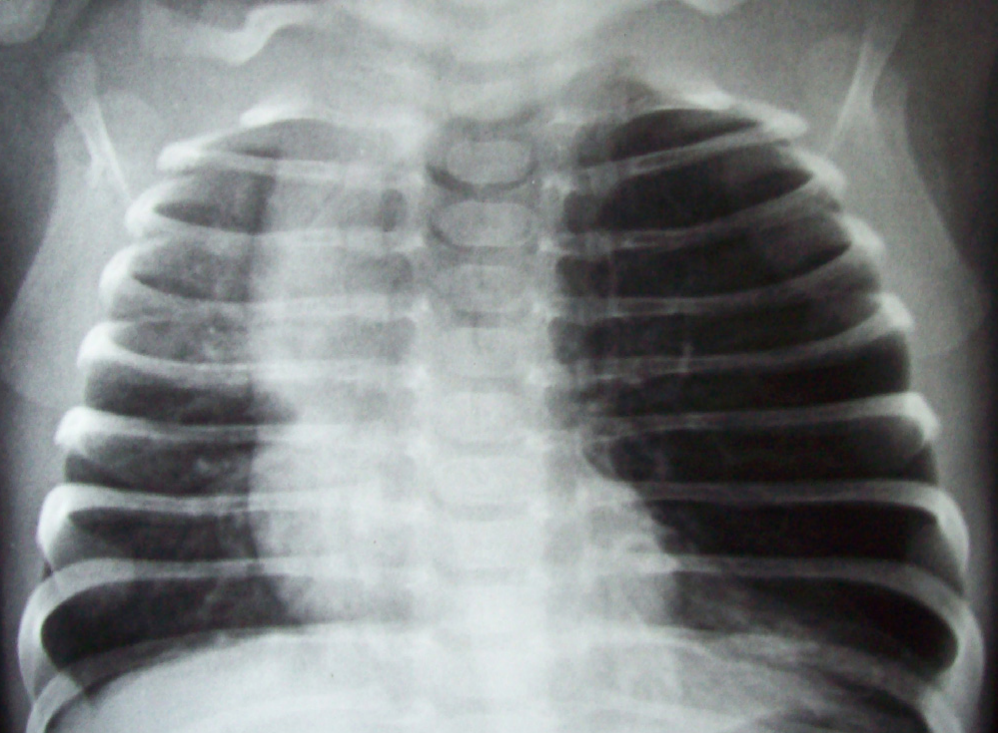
Chest X-ray PA view. Chest X-ray showed marked overdistension of the the left upper lobe with mediastinal shift to the right and collapse of the ipsilateral remaining lung field.
